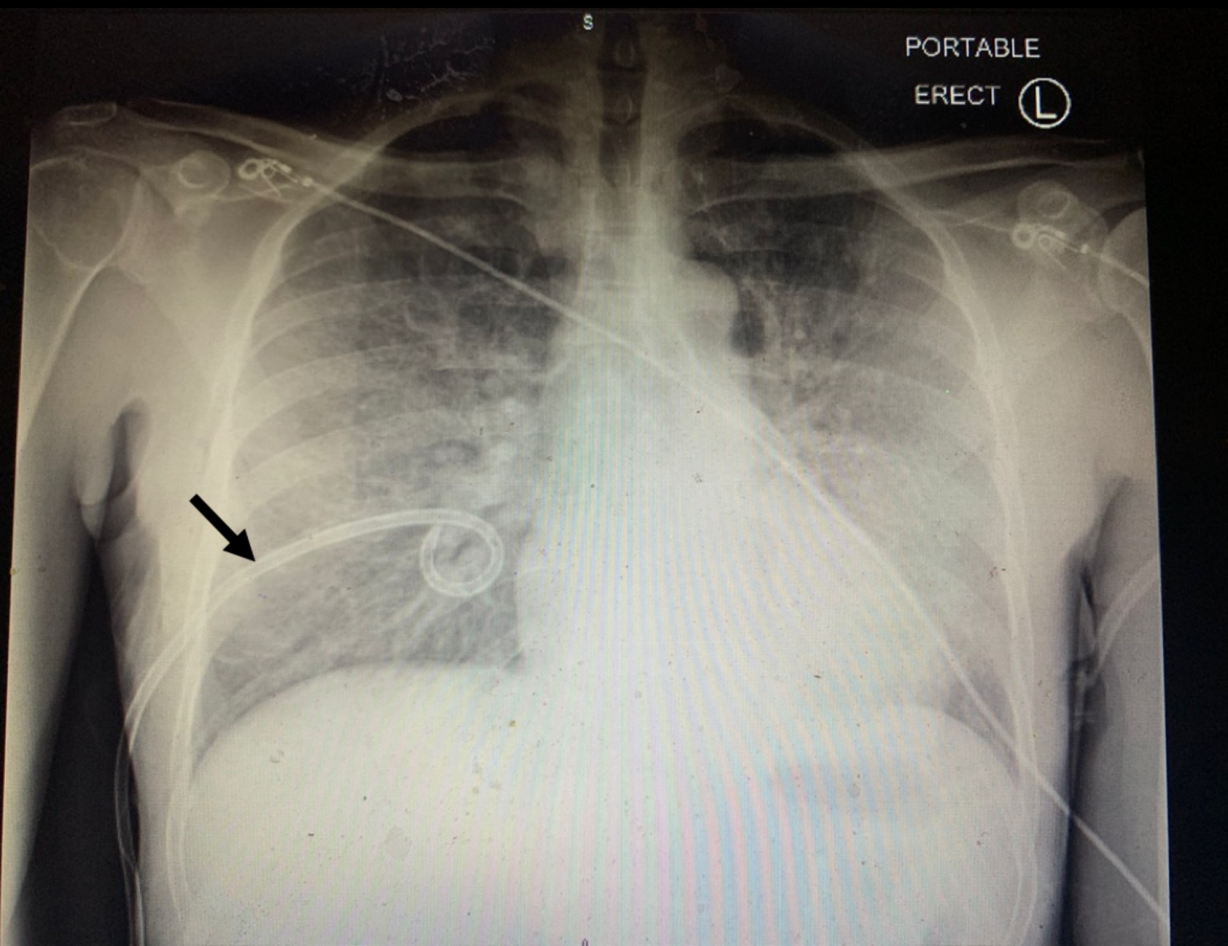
Chest X-ray following the chest tube placement (arrow) showed resolution of the pneumothorax
